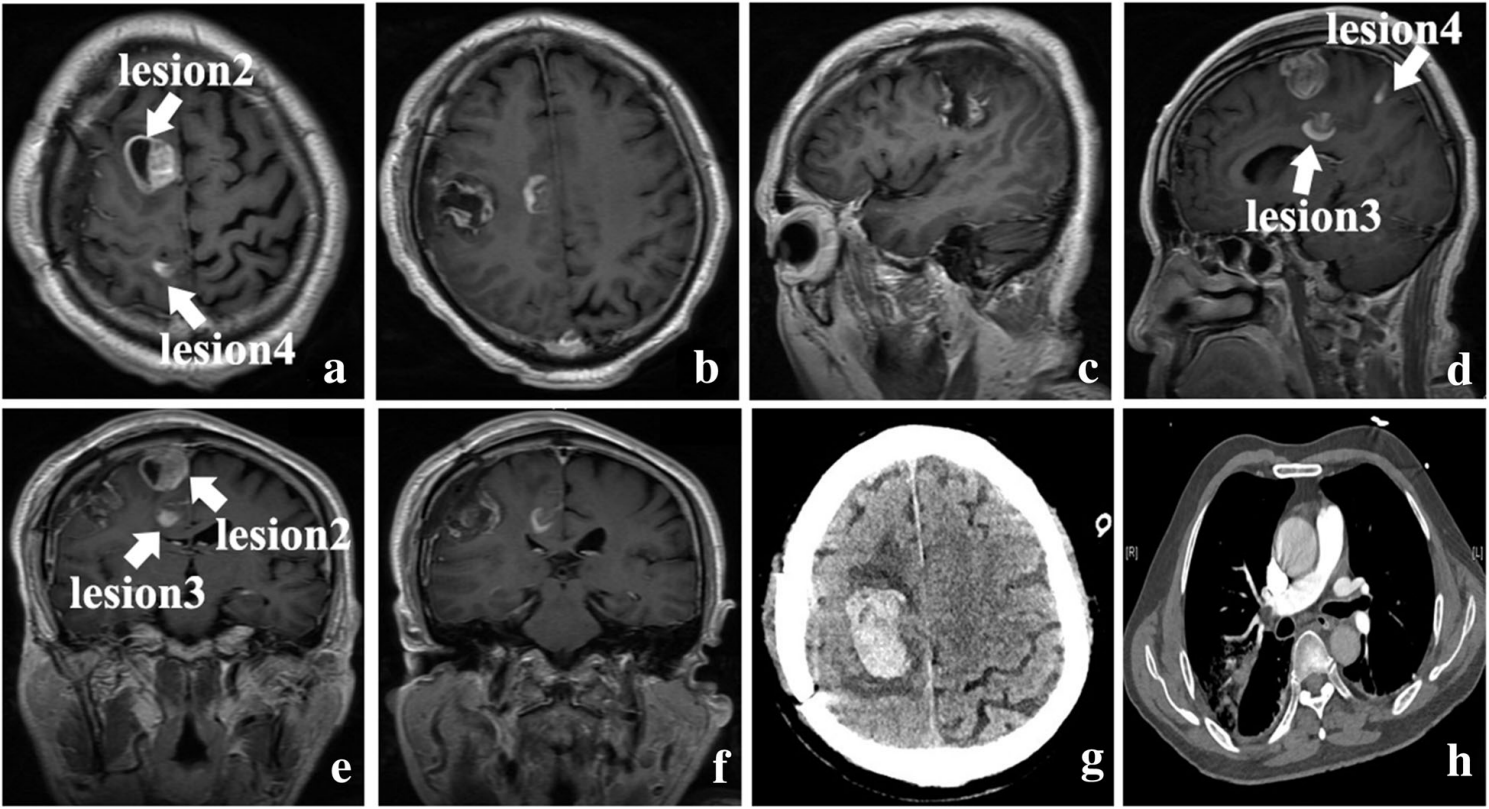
The axial, sagittal, and coronal contrast-enhanced MRI images after surgery (a–f). Emergent CT scan showed hemorrhage of the residual tumor. g The CT angiography of pulmonary artery showed a sign of pulmonary artery embolism (h)
